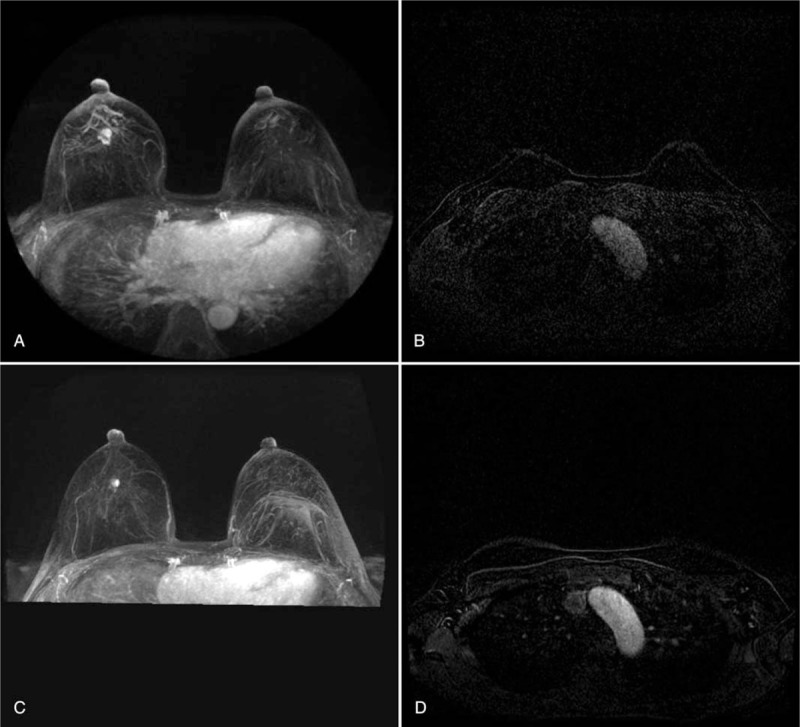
After administration of palbociclib with letrozole, breast MRI as confirmed that the treatment was effective. A and B, Breast MRI after 3 months of administration. C and D, Breast MRI after 11 months of administration. MRI = magnetic resonance imaging.
